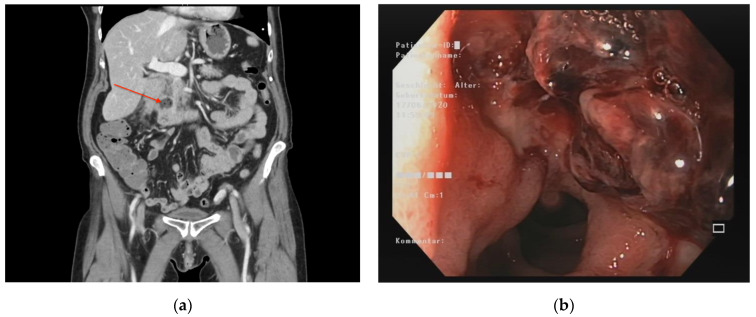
(a) CT scan. In the coronal reconstruction of the portovenous contrast-enhanced phase detection on a perforated duodenal diverticulum (red arrow) as a cause of peritonitis and sepsis. (b) Endoscopy. Chronic peptic duodenal ulcer with penetration into the hepatopancreatic duct.
